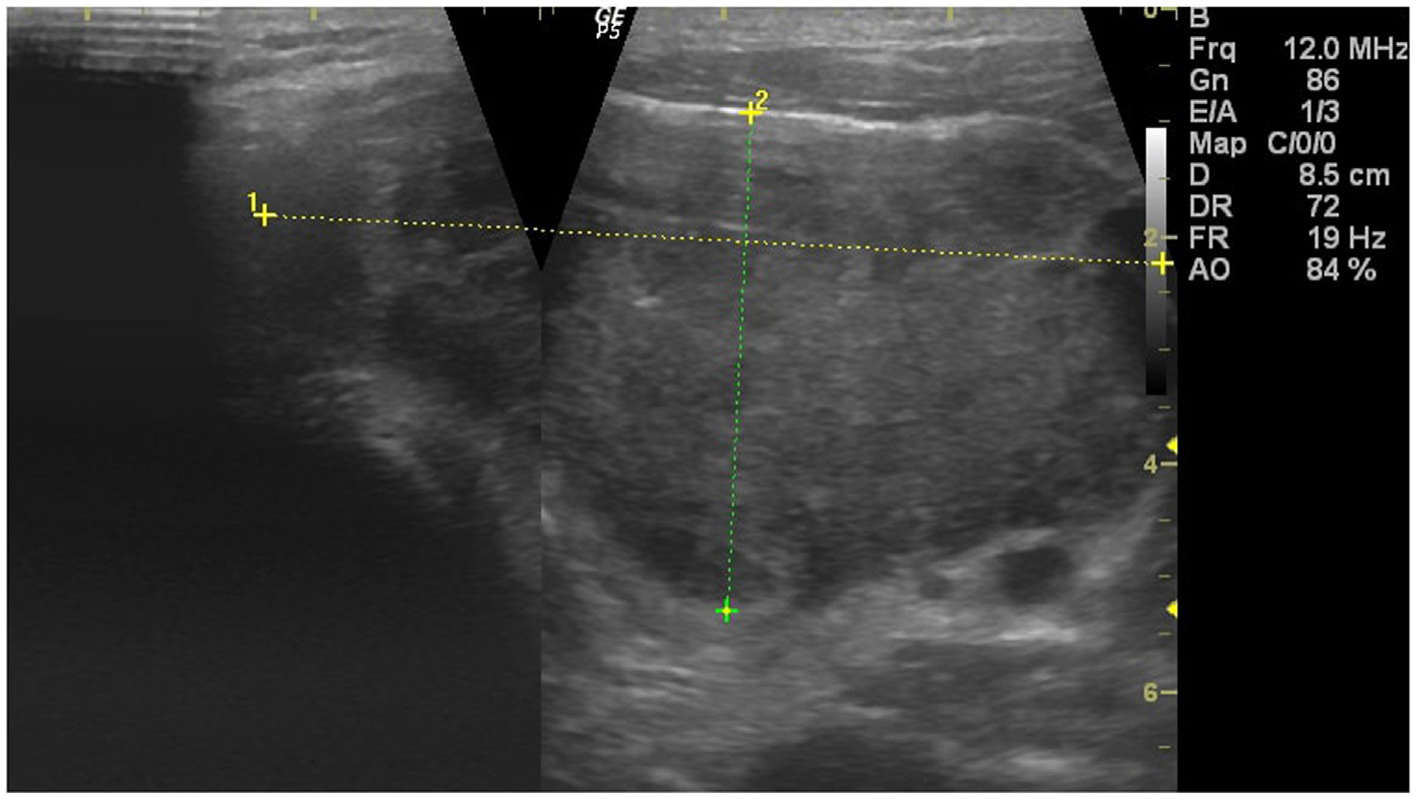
Ultrasound appearance of the tumor.
